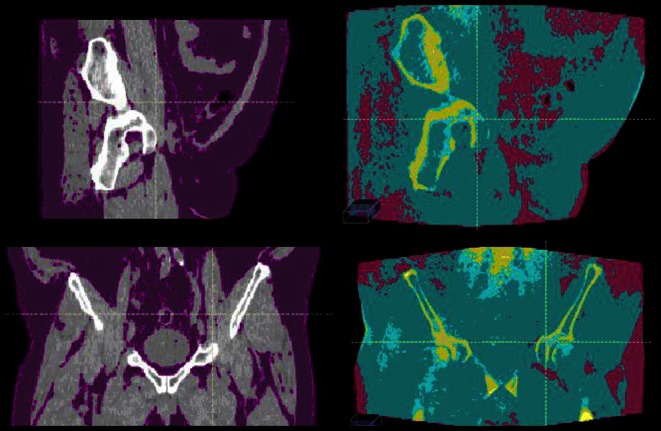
Sagittal (*top row*) and coronal slices (*bottom row*) of a pelvic case with a high proportion of adipose tissue. *From left to right*: the PCT_CBCT_ with tissue density < 0.95 g/cm^3^ colored *purple,* and CBCT with RS_auto_ CTN adjustment. In the CBCT images *purple*, *turquoise*, and *yellow* represent adipose (0.95 g/cm^3^), connective tissue (1.05 g/cm^3^), and bone (1.6 g/cm^3^), respectively. *PCT* planning computed tomography, *CBCT* cone beam CT, *CTN* CT number
